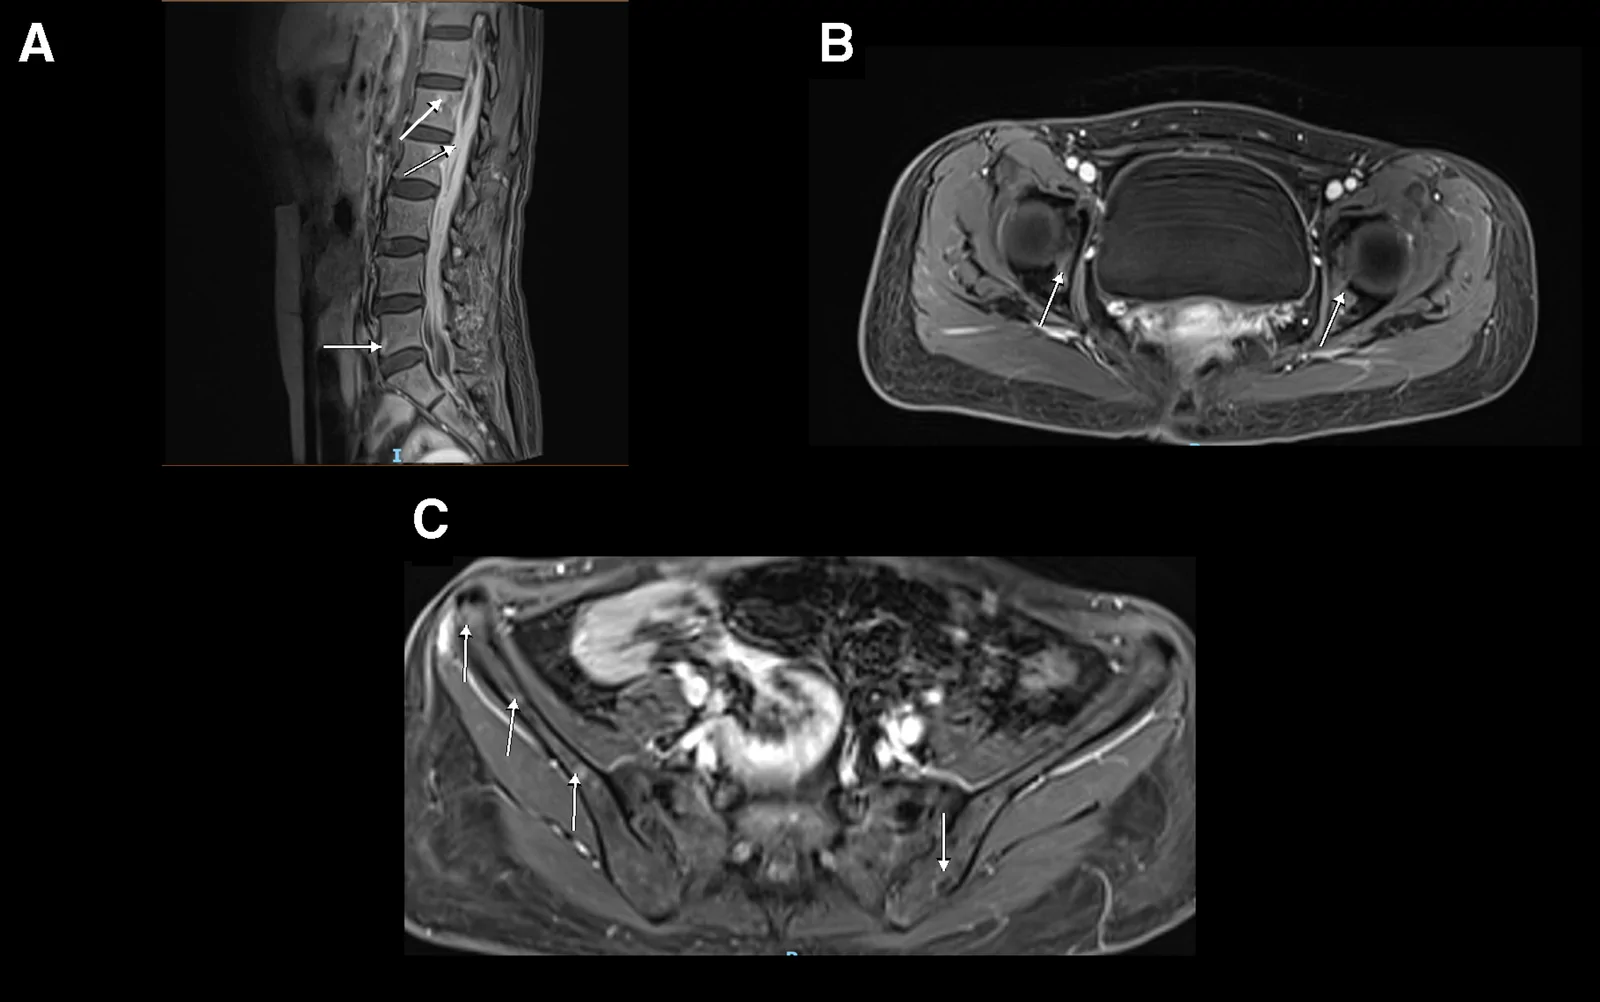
MRI findings before craniospinal irradiation. (A) Sagittal contrast-enhanced lumbar MRI obtained on January 8, 2024, demonstrates representative enhancing vertebral lesions at L1 and L5 and abnormal enhancement involving the filum terminale and cauda equina (arrows). (B) Axial contrast-enhanced pelvic MRI obtained on January 4, 2024, demonstrates representative bilateral acetabular lesions (arrows). (C) Axial contrast-enhanced pelvic MRI from the same examination demonstrates representative bilateral iliac lesions (arrows). The arrows identify representative abnormalities and are not intended to indicate the complete extent of disease. MRI = magnetic resonance imaging.
